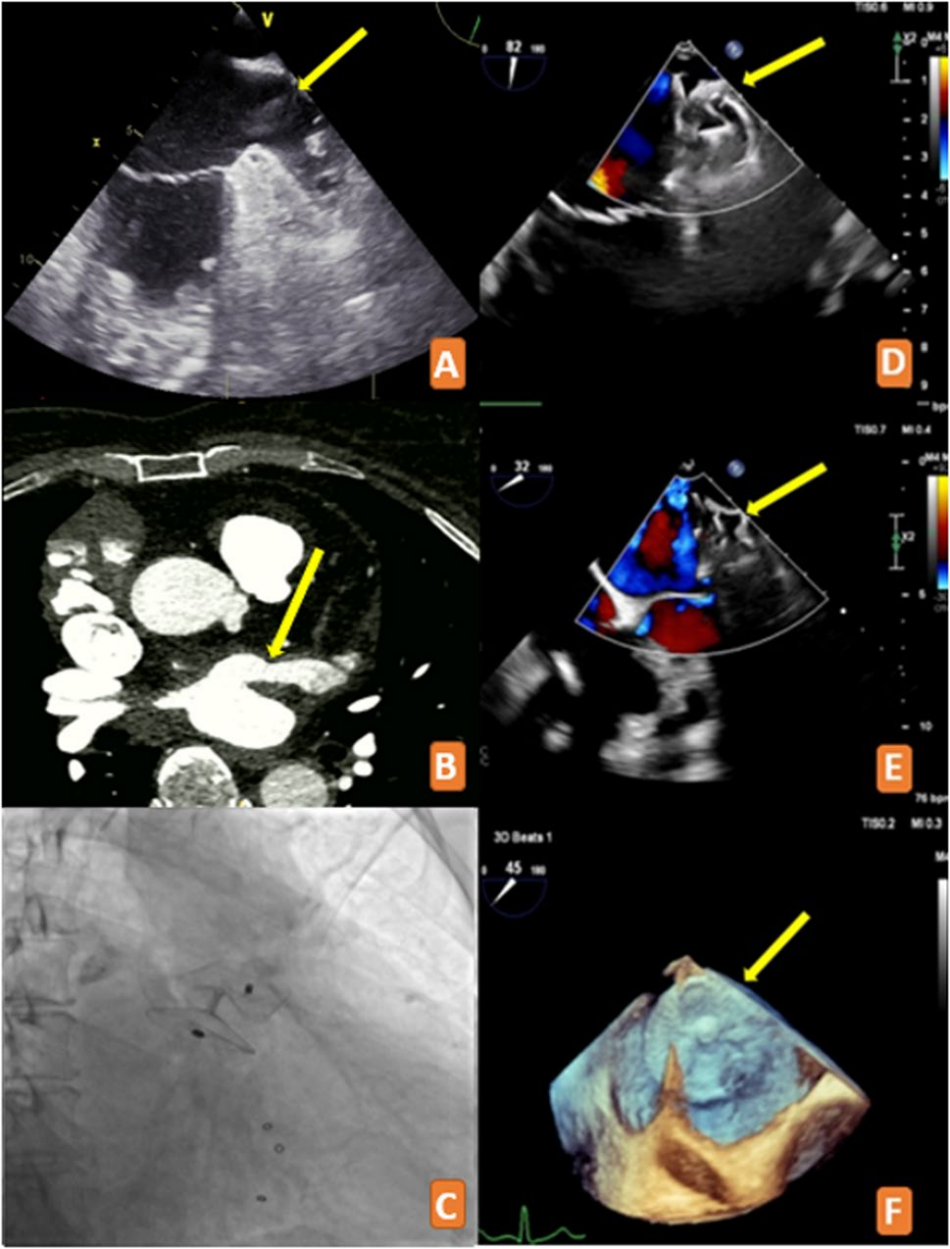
A Pre-surgical transesophageal echocardiography demonstrating thrombus formation at the left atrial appendage ostium. B Cardiac CTA demonstrating 10 mm residual left atrial appendage leak (arrow). C Fluoroscopic image (RAO cranial projection) showing successful device deployment with no contrast passage, confirming complete leak closure. D Transesophageal echocardiography with color Doppler demonstrating no flow passage through the device, indicating successful leak closure. E Two-dimensional transesophageal echocardiography at 2-month follow-up showing no leak passage. F Three-dimensional transesophageal echocardiography demonstrating stable device position
